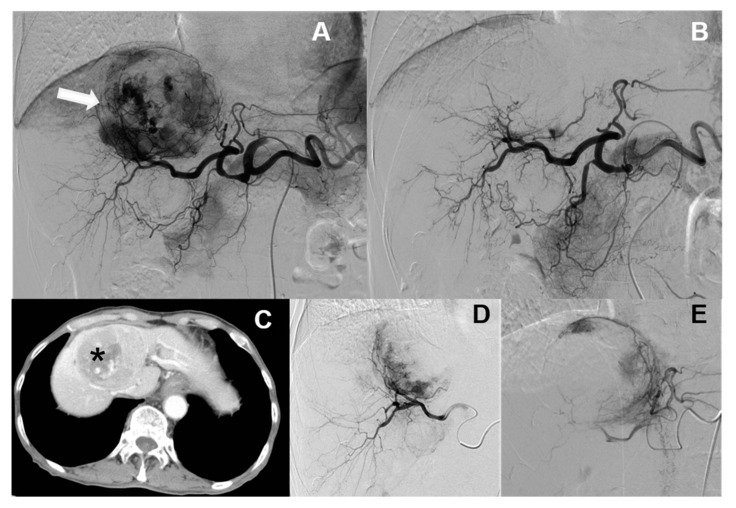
Microsphere embolization (DC bead TACE). A 7.3 cm hepatocellular carcinoma (HCC) with arterial enhancement in segment 4 and 8 of the liver in both angiography (A, white arrows) and CT (C, * star) images. TACE is performed by using drug eluting microspheres (D and E). Follow-up angiography shows complete embolization of the tumor (B).
